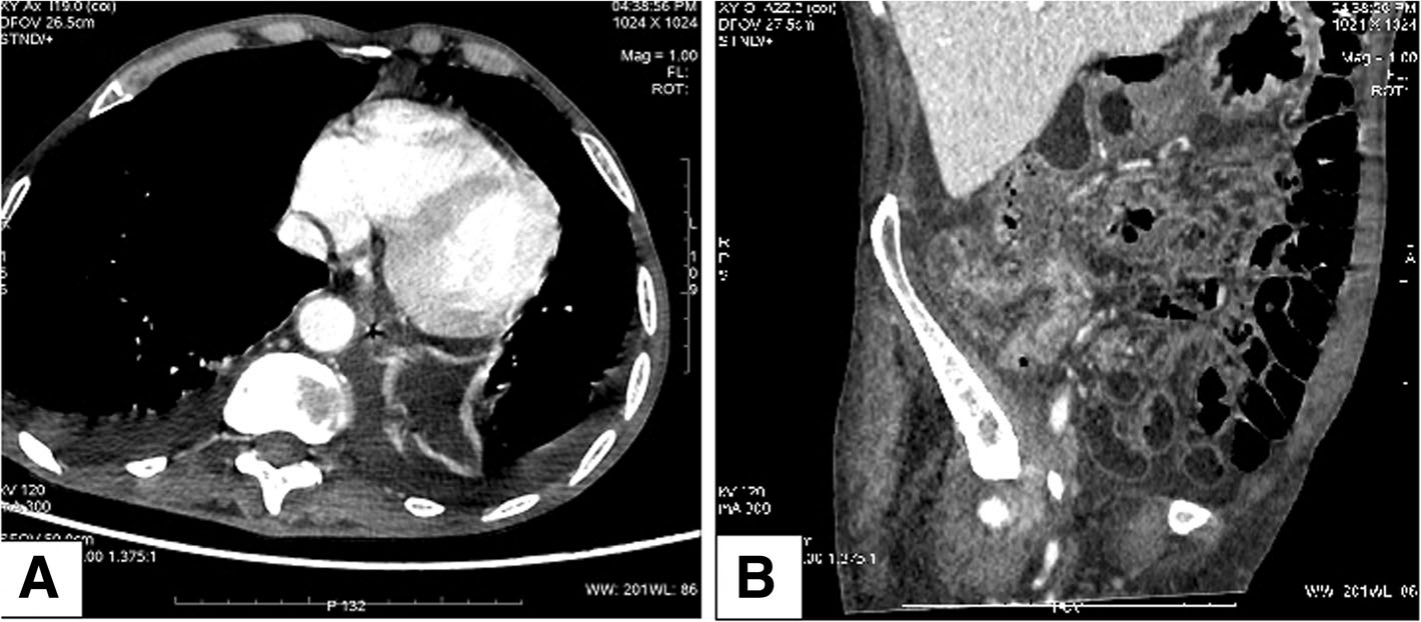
Chest and abdominal enchaned CT revealed polyserositis (pleural effusion, ascites, pericardial effusion) (a) and marked thickening of the entire colonic mucosa (b)
